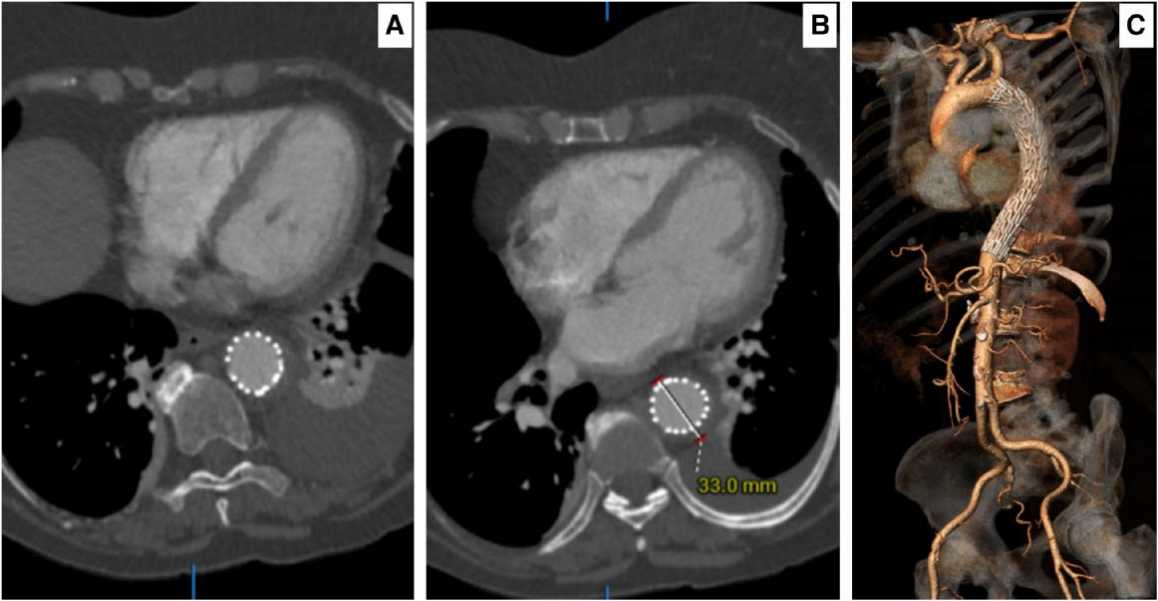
(*A*) A 96 h axial computed tomography angiography post intervention demonstrating total modulation of acute intra-mural haematoma. (*B*) A 96 h axial computed tomography angiography post intervention demonstrating a decrease in acute intra-mural haematoma size to 33 mm. (*C*) A 96 h three-dimensional computed tomography angiography reconstruction demonstrating no stent graft–induced new entry and patent great vessels of head and neck visceral branches with the stable aorta.
